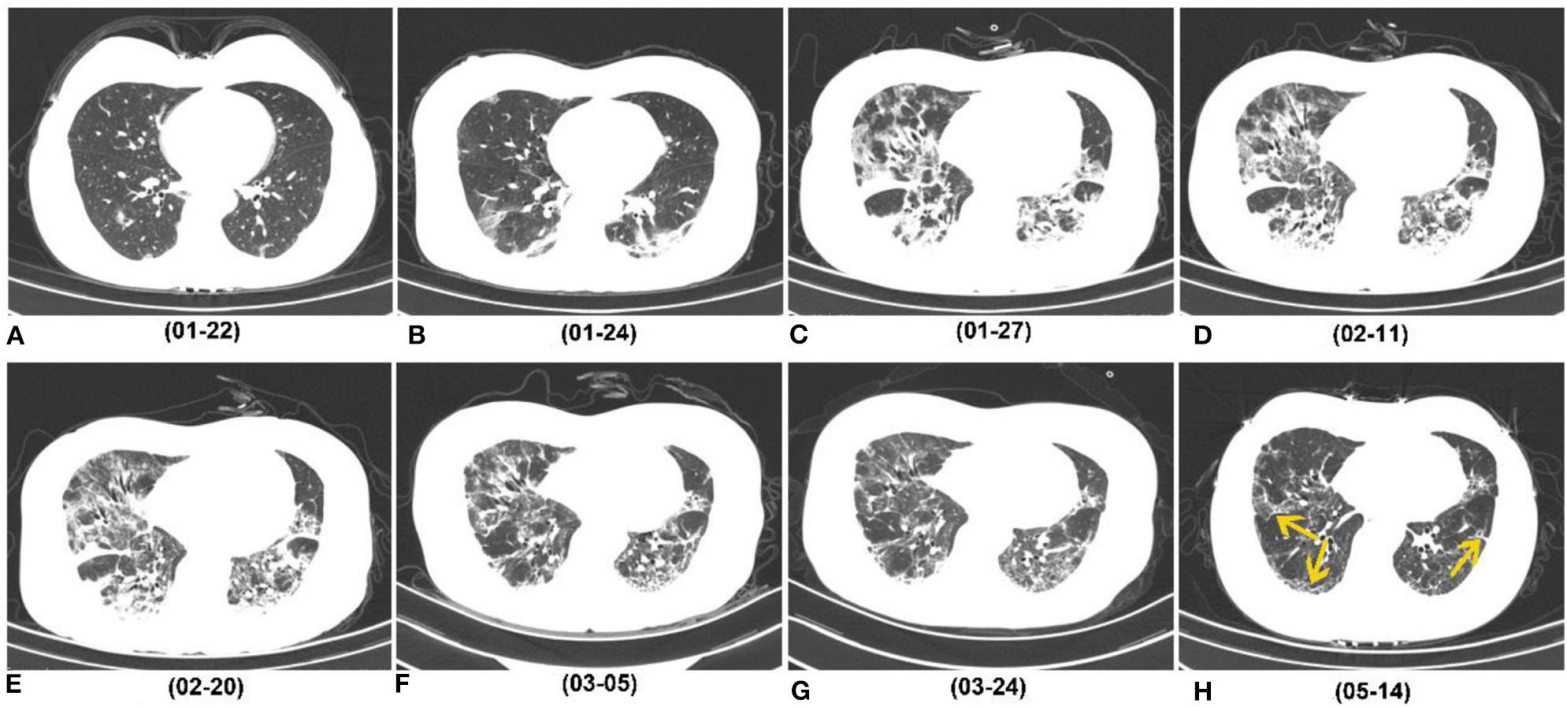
Series of follow-up CTs of a 40-year-old woman with COVID-19 who caught a fever on January 19, 2020 and was admitted at hospital on January 27, 2020. (A) Initial chest CT on January 22 (3 days after symptoms onset) showed sporadic GGO in bilateral lower lobes. (B–E) Two days later, consolidation appeared. Whereafter, the extent of GGO and consolidation enlarged, and reached its peak on February 11, and remained the peak level until February 20. (F,G) At the recovery stage, lesions were gradually absorbed. (H) On May 14 (116 days after symptoms onset), most consolidations were absorbed, while irregular interfaces and parenchymal bands were observed as evidence of fibrotic change (yellow arrow). COVID-19, coronavirus disease 2019; GGO, ground-glass opacity.
